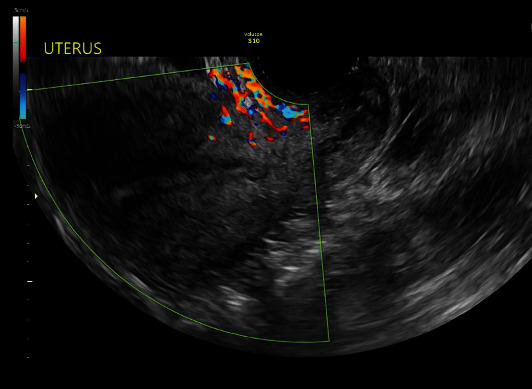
Transvaginal ultrasound performed in clinic for evaluation of abnormal uterine bleeding. Endometrial thickness was noted to be 4.70 mm with a uterine length of 9.1 cm and width of 8.2 cm.
